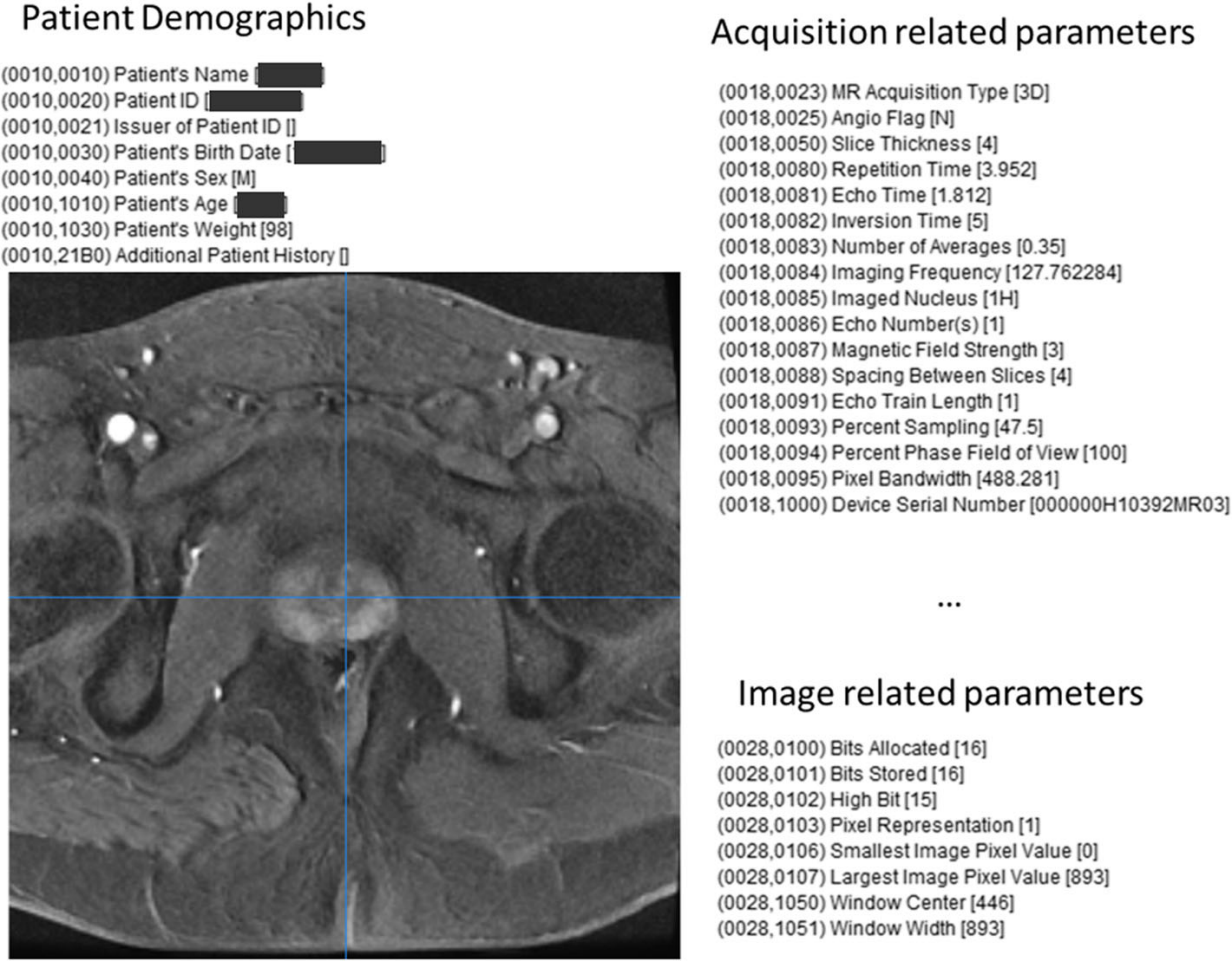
An example of a Digital Imaging and Communications in Medicine (DICOM) contrast-enhanced magnetic resonance image (T1-weighted sequence) of the prostate and DICOM metadata about patient demographics, acquisition-related parameters and image-related parameters
